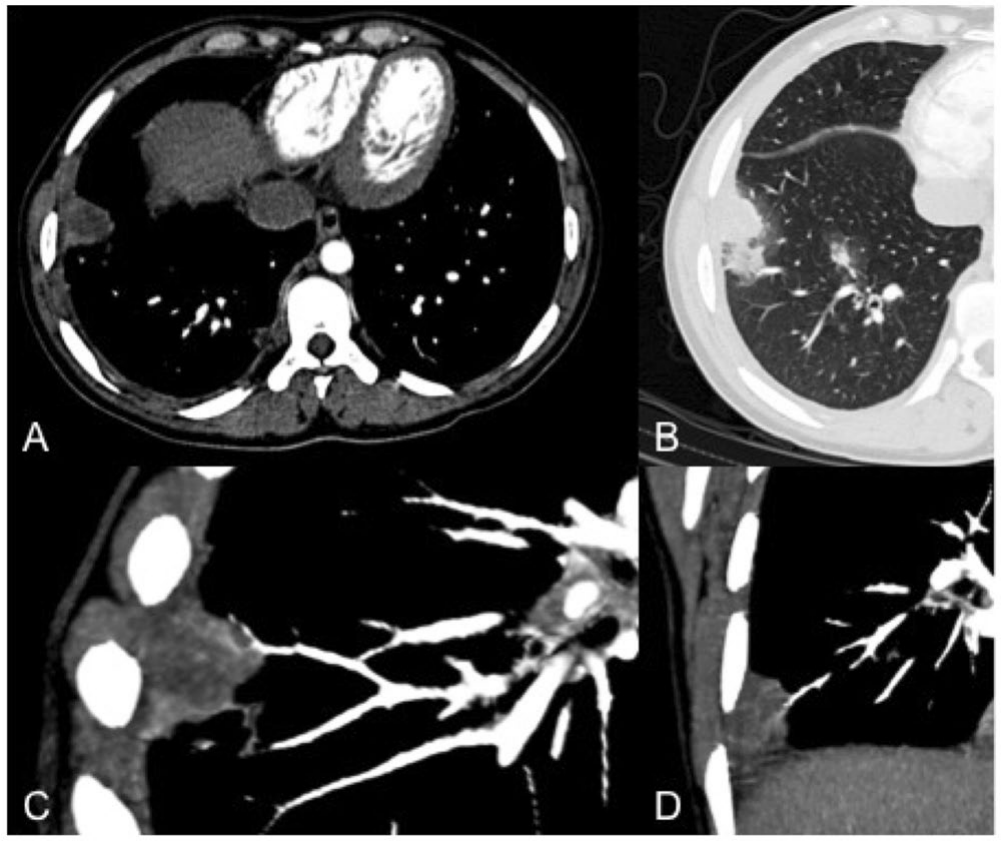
Computed tomography pulmonary angiography (CTPA) study of a 29-year-old male, immunocompromised patient. The infarct shaped subpleural consolidation showed central low attenuation (A) with a surrounding peripheral halo sign (B). CTPA showed vessel interruption within the lesion (C,D).
